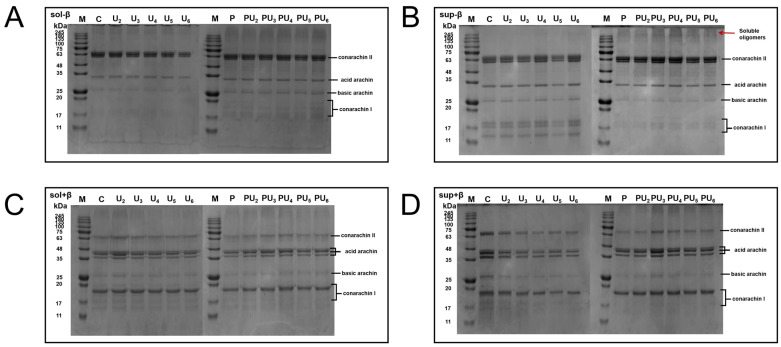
Sodium dodecyl sulfate-polyacrylamide gel electrophoresis (SDS-PAGE) pattern of (A,C) solutions and (B,D) supernatants for native (C) and treated PP with high-intensity ultrasound (HIU, 200–600 W) and pH-shifting (pH 12), alone (U_2_, U_3_, U_4_, U_5_, U_6_, and P) or in combination (PU_2_, PU_3_, PU_4_, PU_5_, and PU_6_) under (A,B) nonreducing and (C,D) reducing conditions. M indicates the marker.
